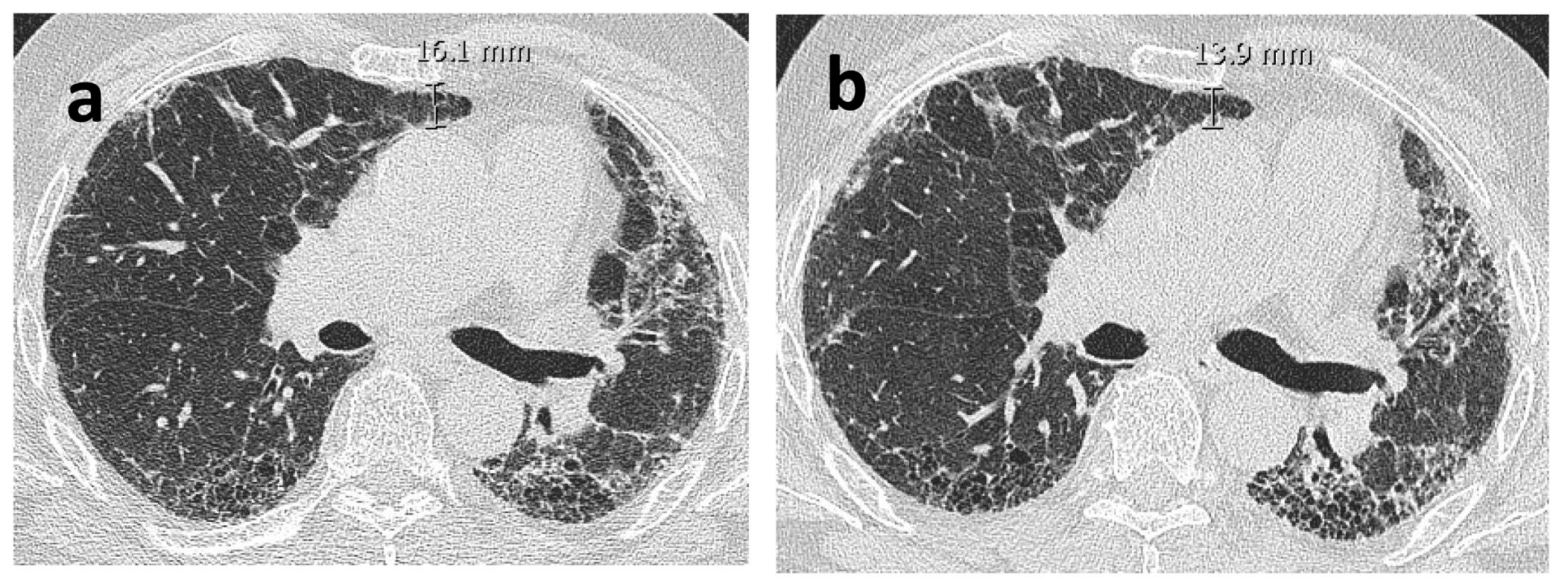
Axial non-contrast enhanced CT images of a 75-year-old male with IPF. Baseline (a) and follow-up (b) images show absence of unequivocal fibrosis progression but a reduction in aortosternal distance, the distance between anterior aspect of ascending thoracic aorta and retrosternum.
